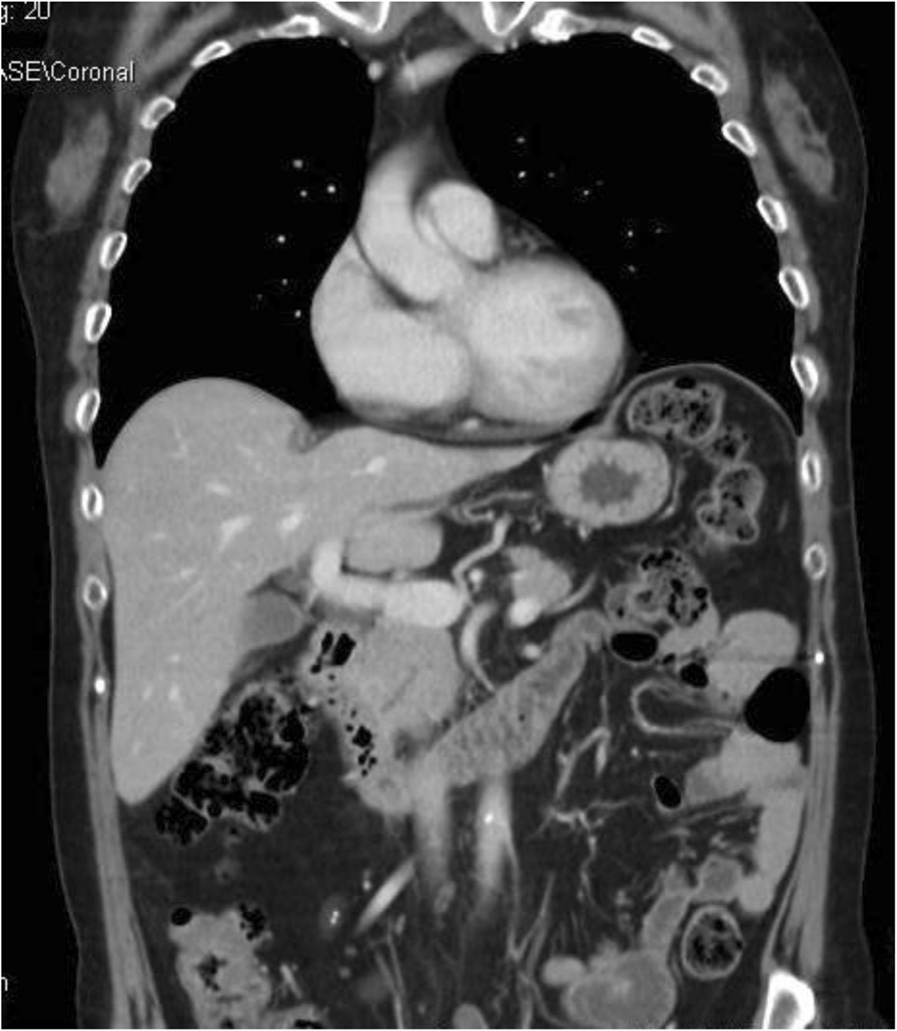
Postoperative imaging. Thorax-abdominal CT scan: normal appearance of the pancreatico-duodenal-biliary region, without any signs of liver or lung involvement
